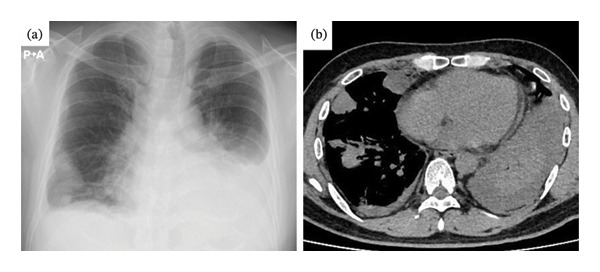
Chest imaging after disease progression. (a) X‐rays showed bilateral pleural effusions predominantly on the left side and increasing pulmonary nodular shadows. (b) CT revealed that each lesion was enlarged and the left lower lobe was collapsed due to pleural effusion.
